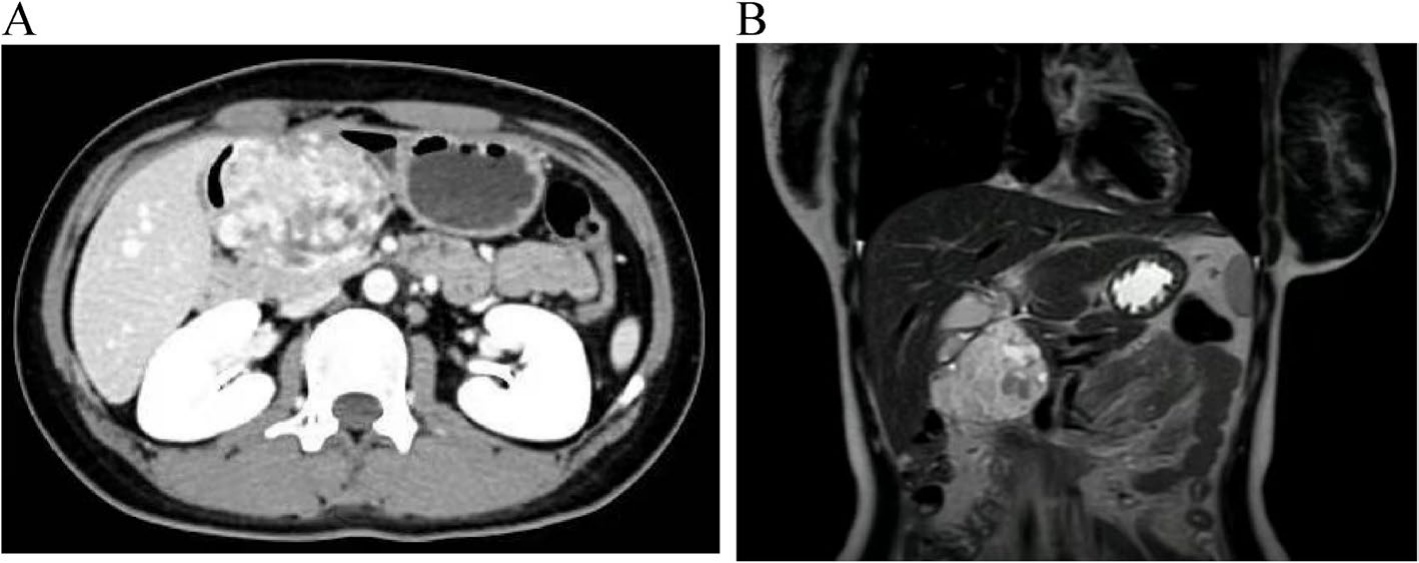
Imaging examination A Abdominal CT shows a mass in the pancreatic head area, the boundary was clear, and the boundary between the lesion and the pancreatic head was not clear. B Abdominal MRI showed a solid mass in the pancreatic head, with clear boundary, and internal necrosis and sac
